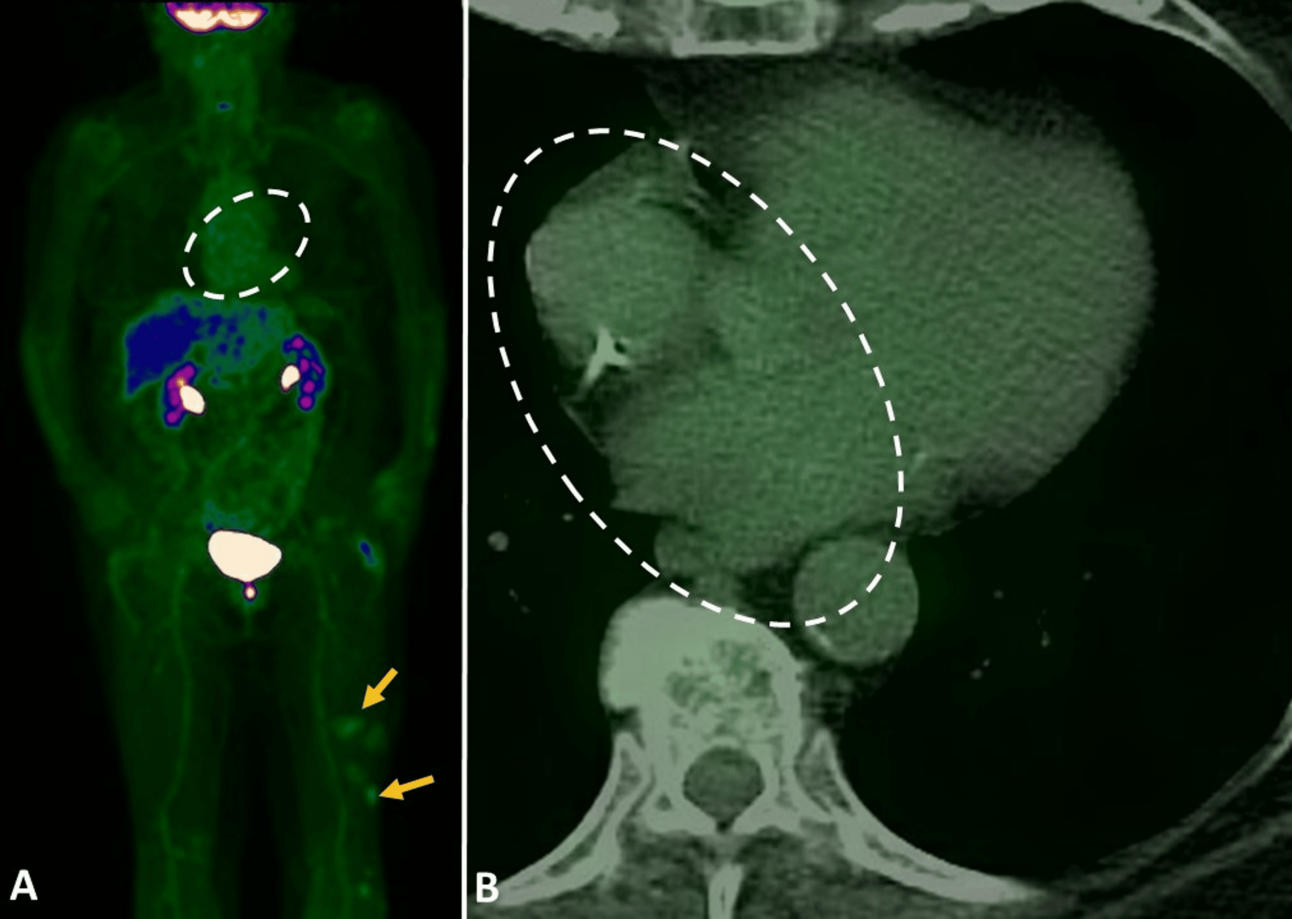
One-year follow-up 18F-FDG PET/CT images (A) maximum intensity projection and (B) transaxial fused PET/CT images demonstrate resolution of biatrial FDG uptake (dashed open oval circle in A and B). Mild foci of FDG uptake in the left thigh (yellow arrows in A) from cutaneous follicular-type diffuse large B-cell lymphoma (DLBCL).
